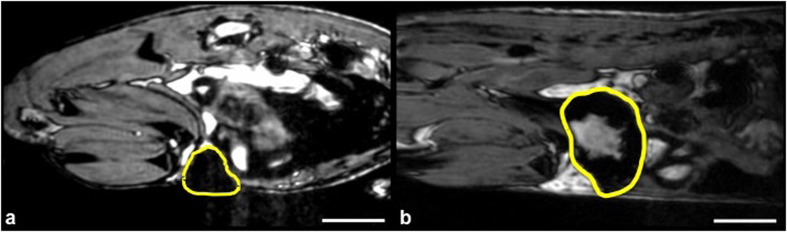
Iron-based MRI cell tracking. Sagittal bSSFP images of mammary fat pad tumors acquired 24 hours post USPIO administration. (a) 4 days post 4T1 cancer cell injection: Signal loss encompasses the entire tumor volume (b) 3 weeks post 4T1 cancer cell injection: The tumor is larger; signal loss is now seen around the periphery of the tumor and not within the central core. Scale bars represent 5 mm.
